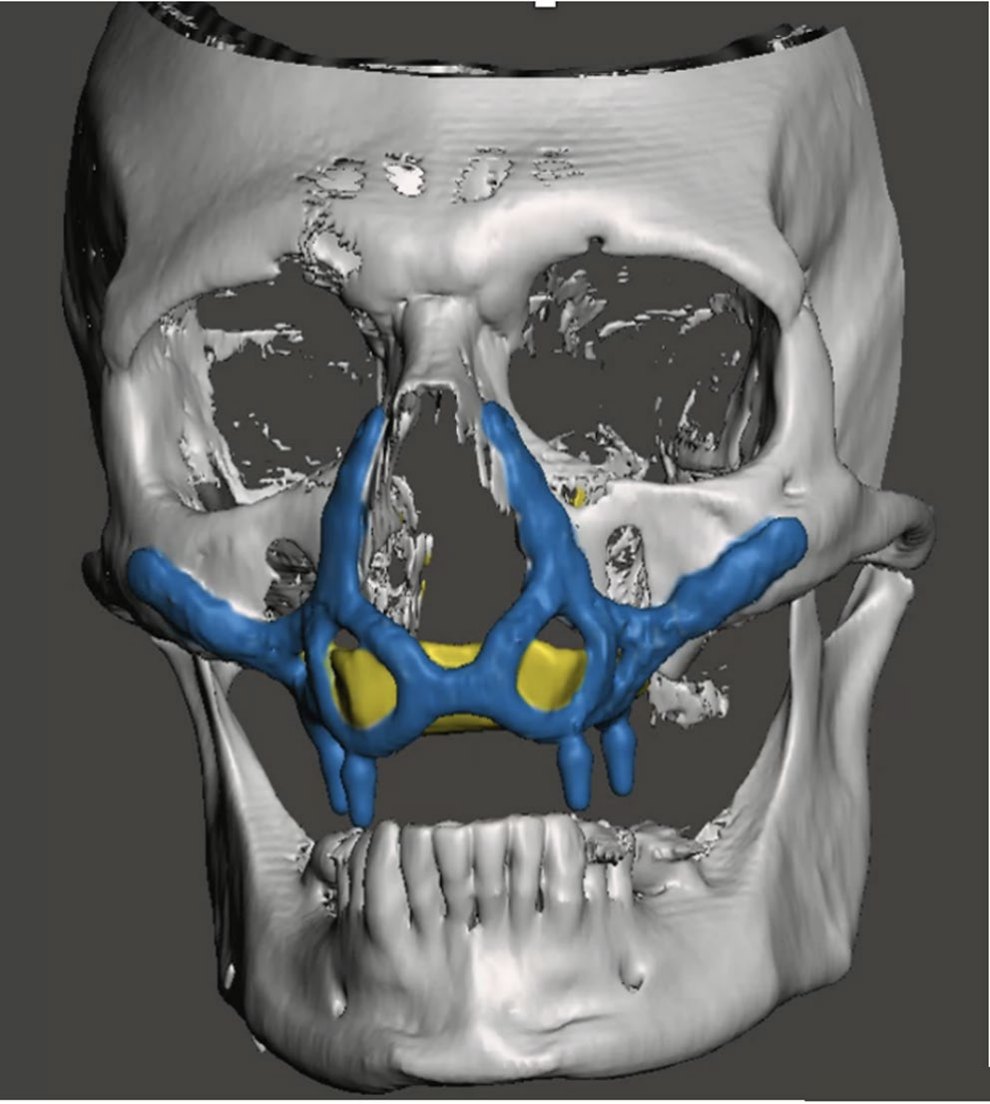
Post-operative 3D CT scan one year after the surgery
